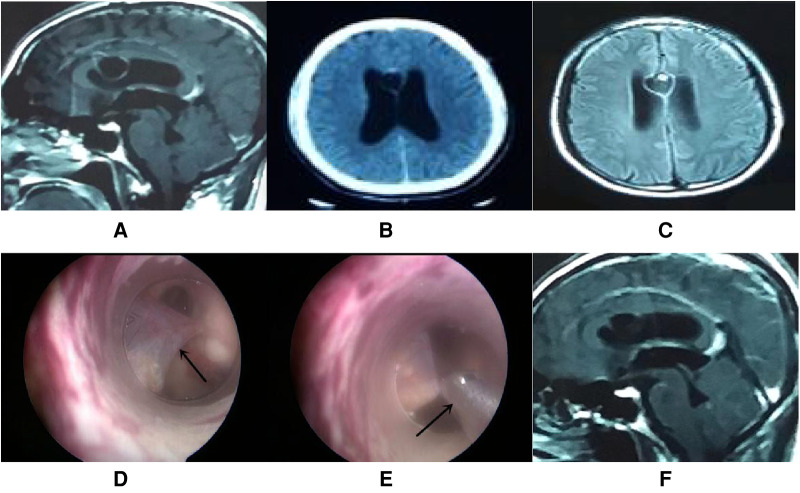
Endoscopic intraventricular surgery was performed to remove a cystic lesion and perform ETV in case 1. (A–C) CT and MRI scans showing a cystic lesion with moderate enhancement in the septum pellucidum with a large ventricle. (D) Surgical image showing the cystic lesion (arrow point) in the septum pellucidum and connected to the ependyma overlying the caudate. (E) Surgical image showing how nasal forceps (arrow point) were used to remove the cystic lesion. (F) MRI scan showing that the enhanced cystic lesion had disappeared by day 5 postoperatively.
